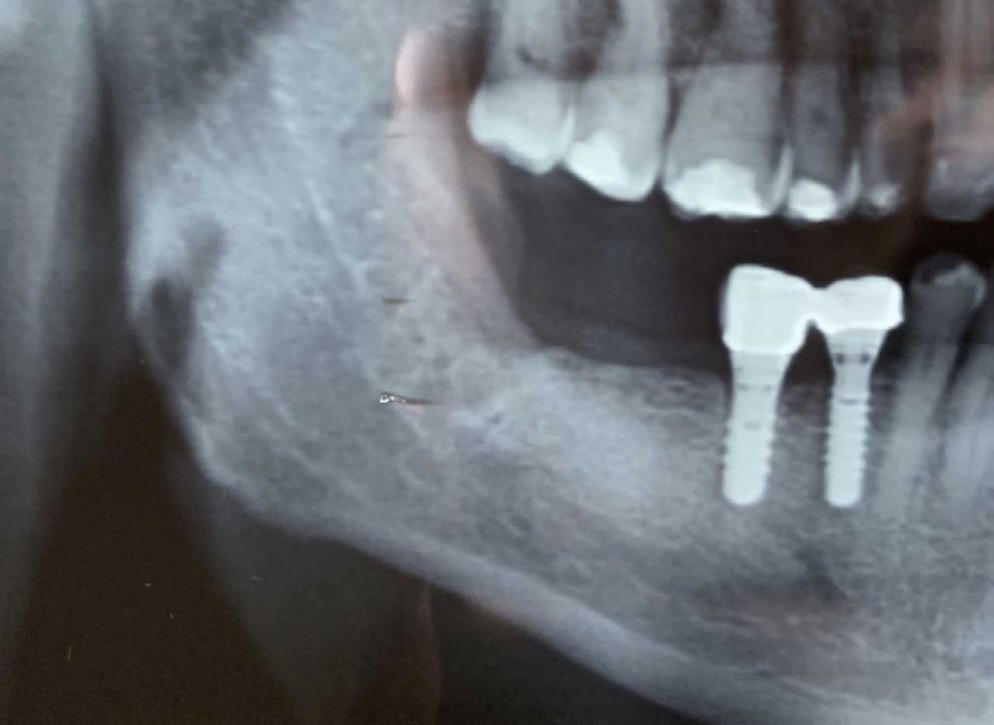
Radiographic appearance at OPT after 6 mo of follow‐up, showing complete bone healing
